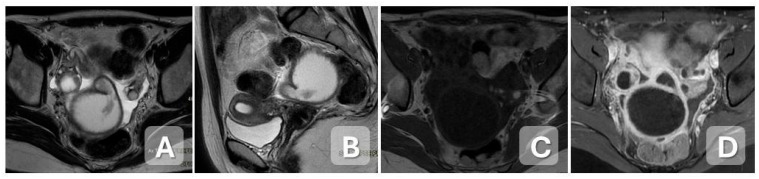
Pelvic inflammatory disease with peritonitis in a 31-year-old woman with a history of endometriosis and recent oocyte retrieval, presenting with pelvic pain and fever 5 days post-procedure. (A,B) Axial and sagittal T2-weighted MR images show a serpiginous left adnexal lesion with corpuscular fluid content, consistent with a dilated and inflamed fallopian tube. (C,D) Axial T1-weighted images before contrast administration and T1-weighted with fat saturation after contrast administration demonstrate marked enhancement of the thickened tubal walls. Associated free pelvic fluid and inflammatory stranding are consistent with secondary peritonitis.
